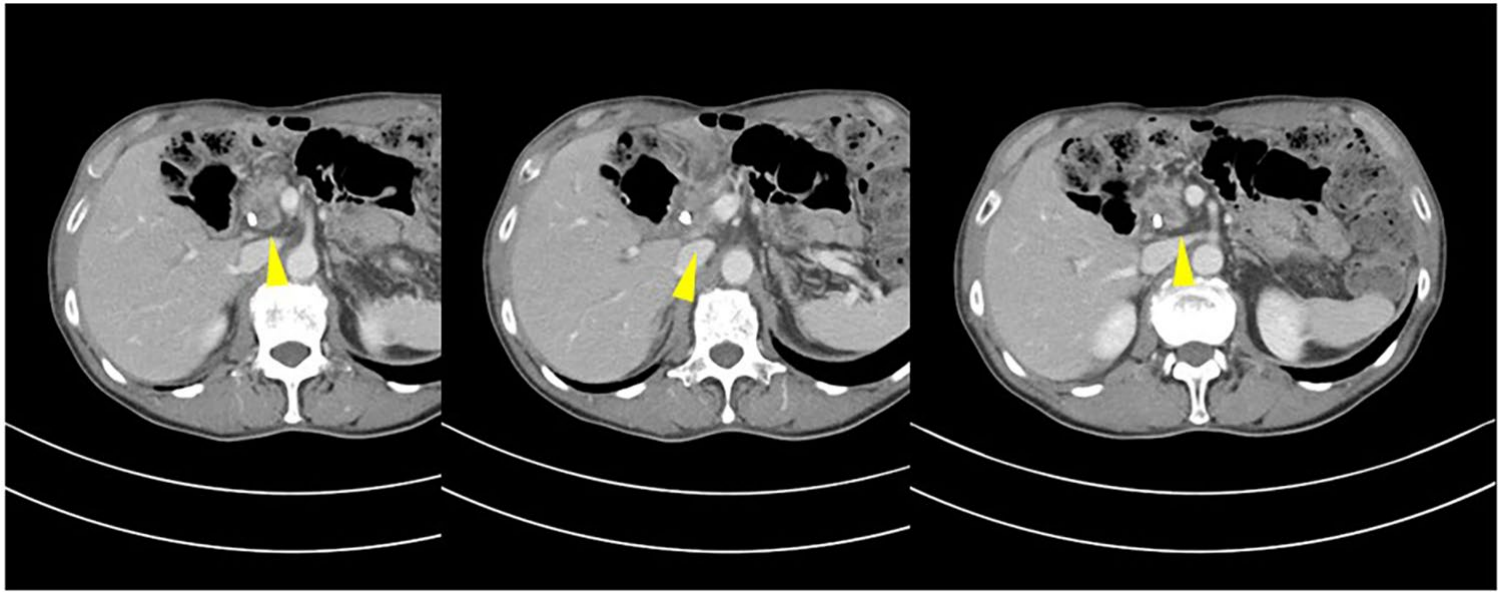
Abdominal contrast-enhanced computed tomography (portal phase). A hypoattenuated tumor with indistinct borders is visible in the pancreatic head. The tumor is in contact with the portal vein at < 180 degrees without vein contour irregularities. Arrowheads indicate pancreatic head tumor
